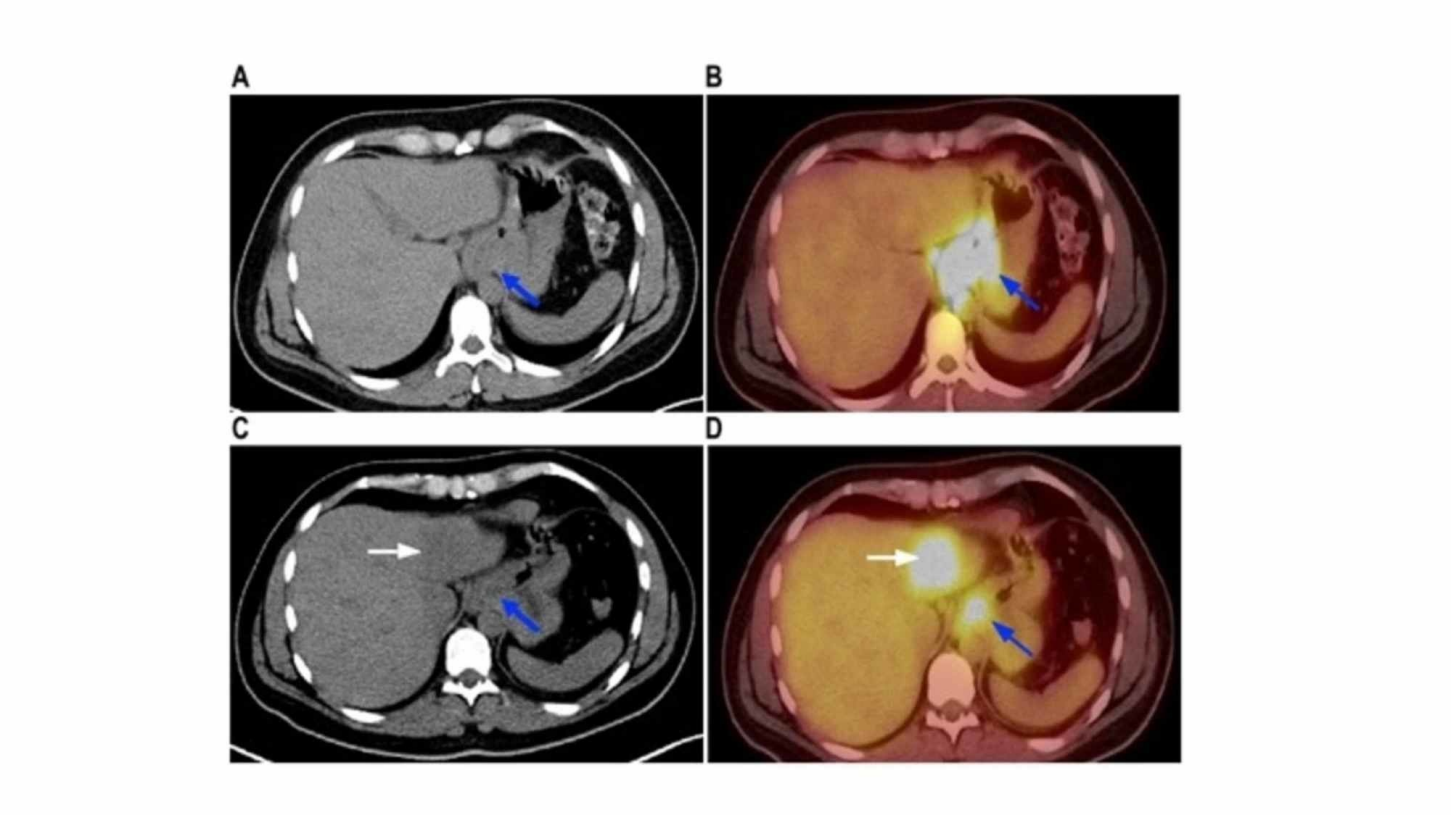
Pre- and post-treatment PET/CT scans. Pretreatment positron emission tomography/computed tomography (PET/CT) scan (panels A and B) show gastroesophageal junction (GEJ) mass and hypermetabolic lesion (blue arrow). PET/CT after completion of neoadjuvant treatment show a faint line of demarcation and hypermetabolic lesion in the left hepatic lobe (white arrow, panels C and D). A decrease in the hypermetabolic activity of the GEJ tumor is noted (blue arrow, panel D).
